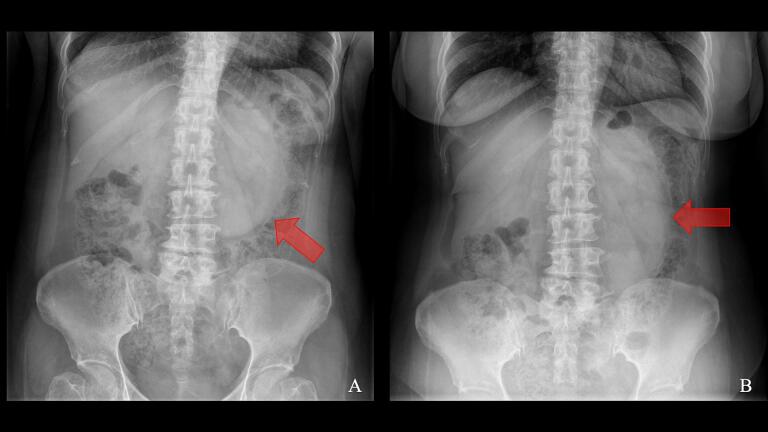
Abdominal X-ray findings in left paraduodenal hernia. A: Supine view, B: Upright view. Abdominal X-ray demonstrating a circumscribed soft tissue mass in the upper quadrant (arrows), displacing the transverse colon inferiorly.
